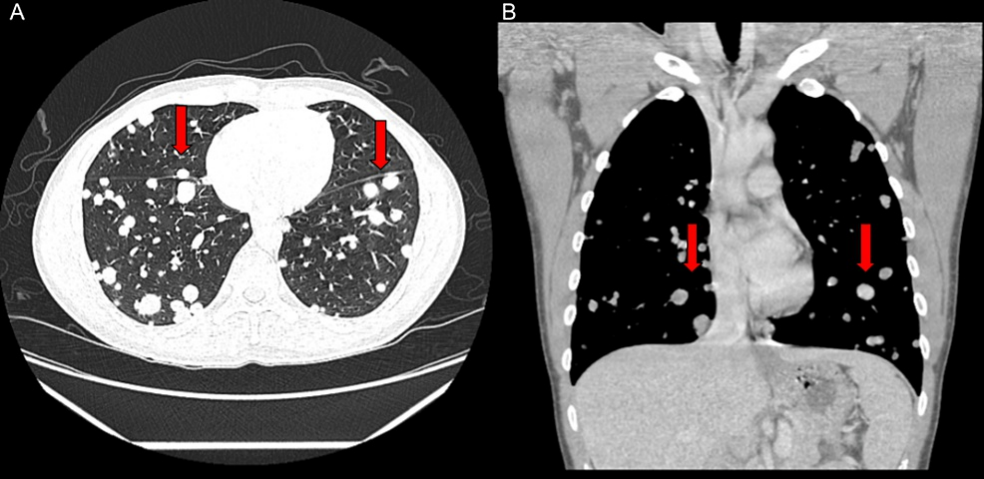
Contrast-enhanced CT scan of the chest in axial (A) and coronal (B) planes demonstrating innumerable pulmonary nodules (red arrows).
